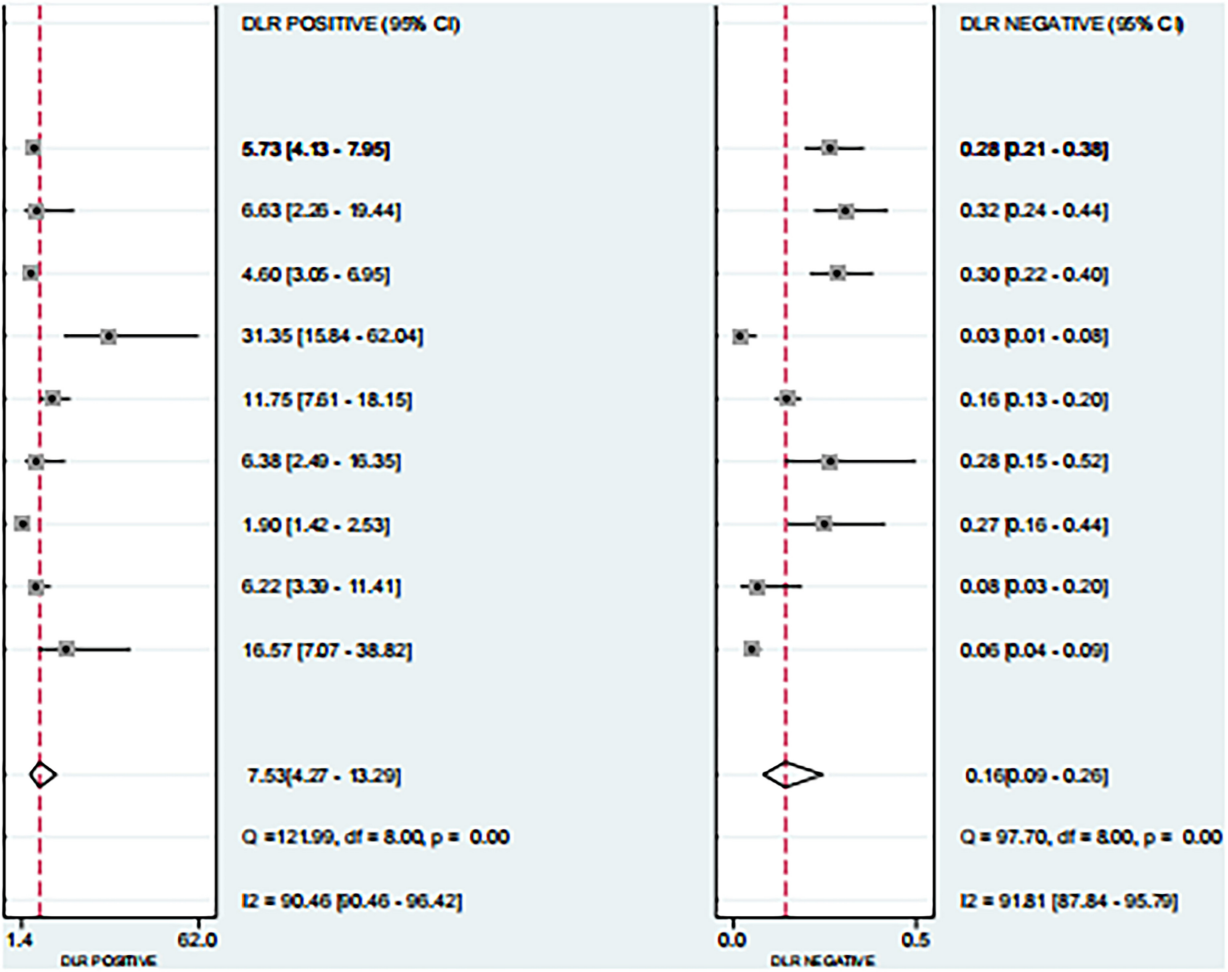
Fagan nomogram for evaluating the clinical utility of 3D transvaginal ultrasound in diagnosing intrauterine adhesions.
